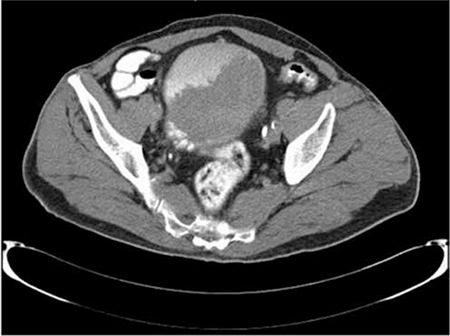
Abdominal computed tomography showing mass in the bladder
